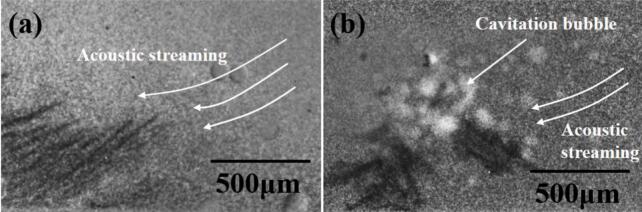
Two typical X-ray images, showing (a) the Ga-40wt.% In dendrites and the acoustic streaming flow (30 W case, see the corresponding Videos 1), (b) the Ga-40wt.% In dendrites and acoustic streaming flow as well as the cavitation bubbles (45 W case, see the corresponding Video 2).
